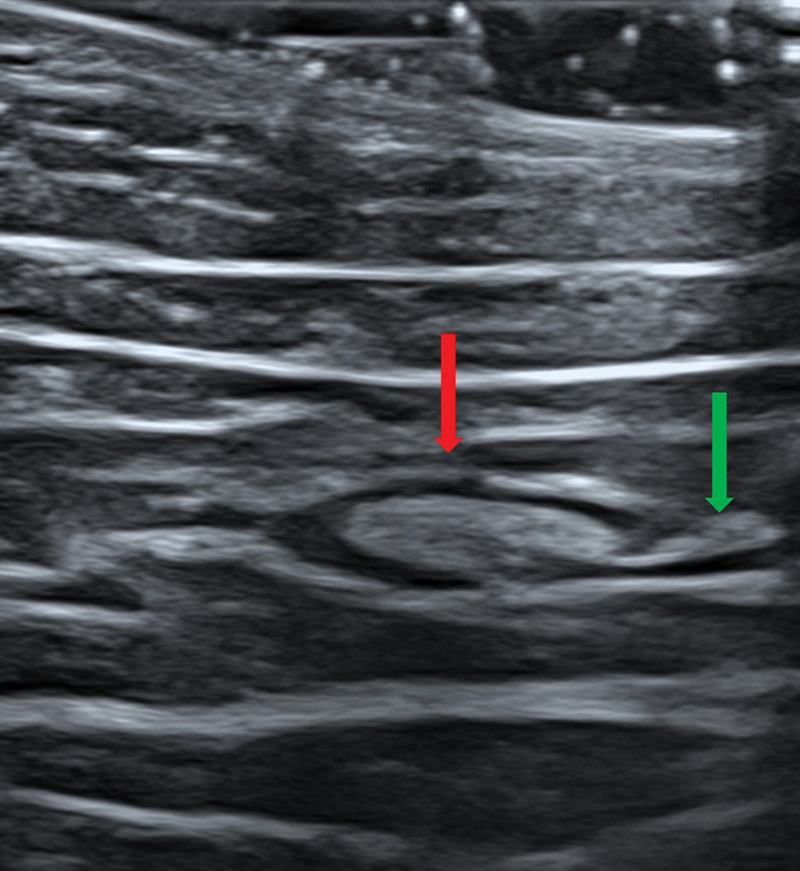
Ultrasonography of a lymph node (groin). A 22 MHz hockey stick probe was used. The red arrow shows the lymph node. The green arrow shows the efferent lymphatic vessel.
